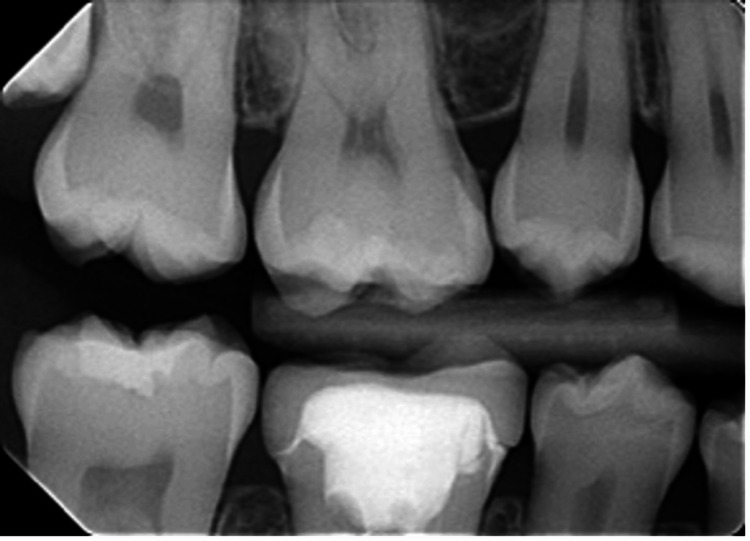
Bitewing radiograph after post crown cementation.
